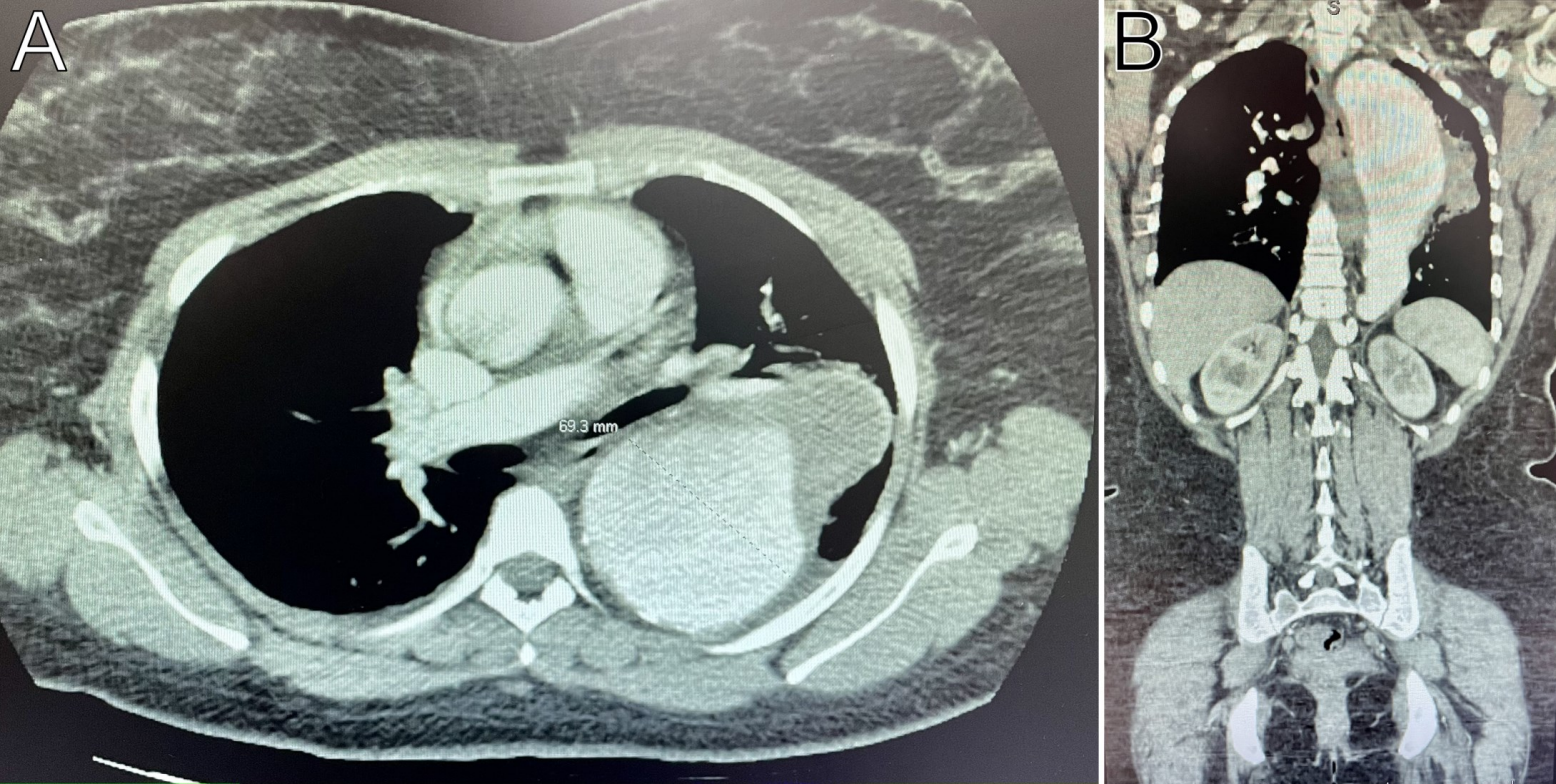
Computed tomography angiogram of the aorta showing a descending thoracic aortic aneurysm and contained aortic rupture. A) the aneurysm in the axial plane, B) the aneurysm in the coronal plane.
